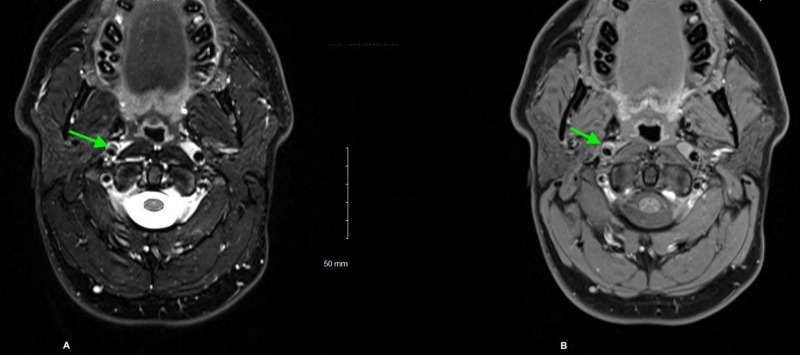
T1 axial sections of MRI neck showing a double lumen abnormality at the petrous segment of the right internal carotid artery (A) and heterogenous signal with contrast enhancement (B) suggesting right internal carotid artery dissection MRI: Magnetic resonance imaging.
